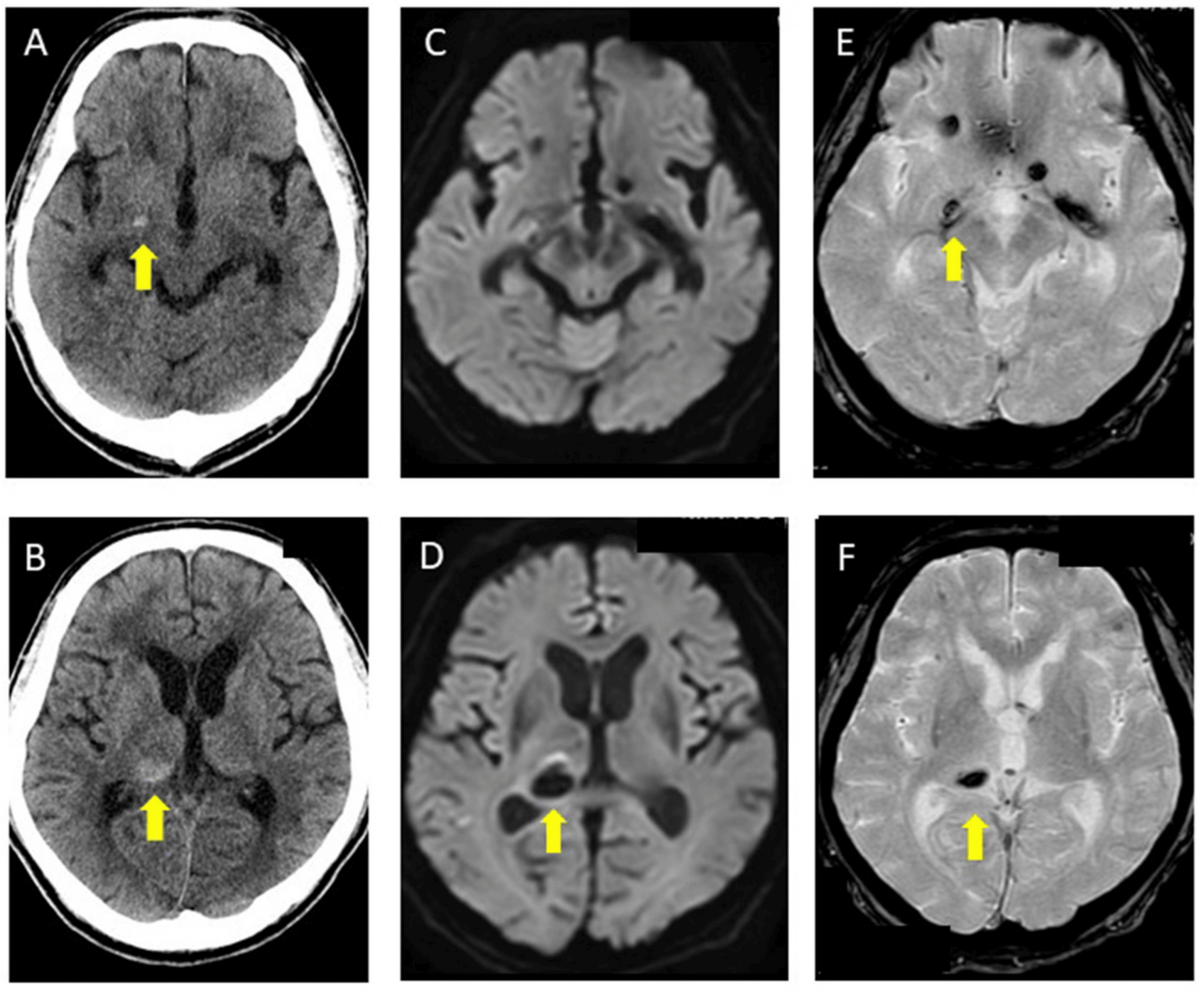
Brain computed tomography (CT), magnetic resonance imaging diffusion-weighted imaging (DWI), and T2* imaging on day 172 A, B: Brain CT showed an asymptomatic thalamic hemorrhage (yellow arrow). C, D: Brain MRI-DWI showed an asymptomatic thalamic hemorrhage (yellow arrow). E, F: T2* imaging showed an asymptomatic thalamic hemorrhage (yellow arrow).
